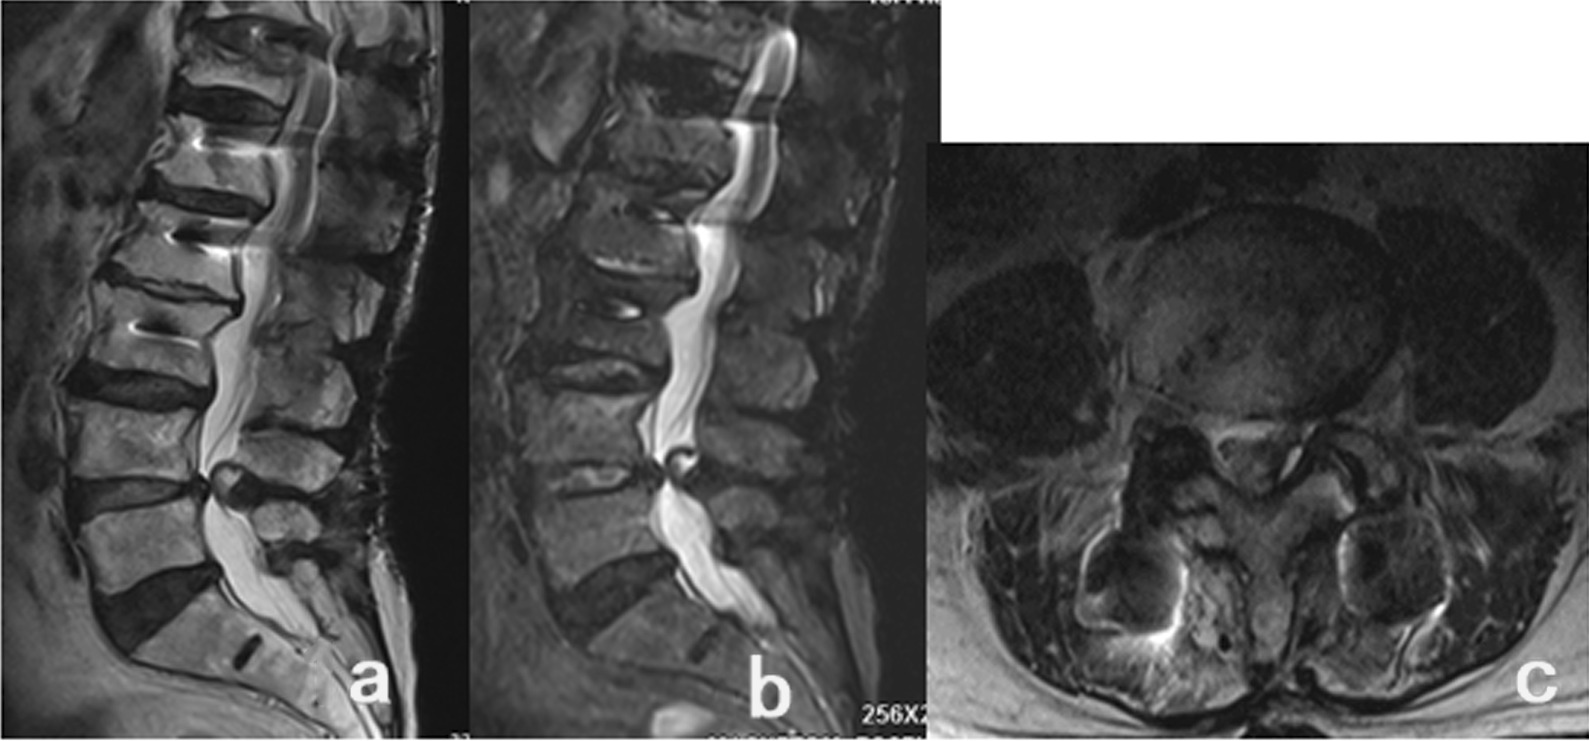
Preoperative MRI showed a mass in the left front of the dural sac in the L4/5 spinal canal a Sagittal T2WI showed spinal stenosis at the L4/5 level, and the mass in the spinal canal showed high signal compared with cerebrospinal fluid. b Sagittal fat compression imaging showed that the signal in the mass was the same as that of the cerebrospinal fluid, showing a high signal, with a clear boundary with the dura mater. c Coronal T2WI showed a mass in the left front of the dural sac in the L4/5 spinal canal, the density was similar to the cerebrospinal fluid, showing a high signal, and a clear boundary with the dural sac
